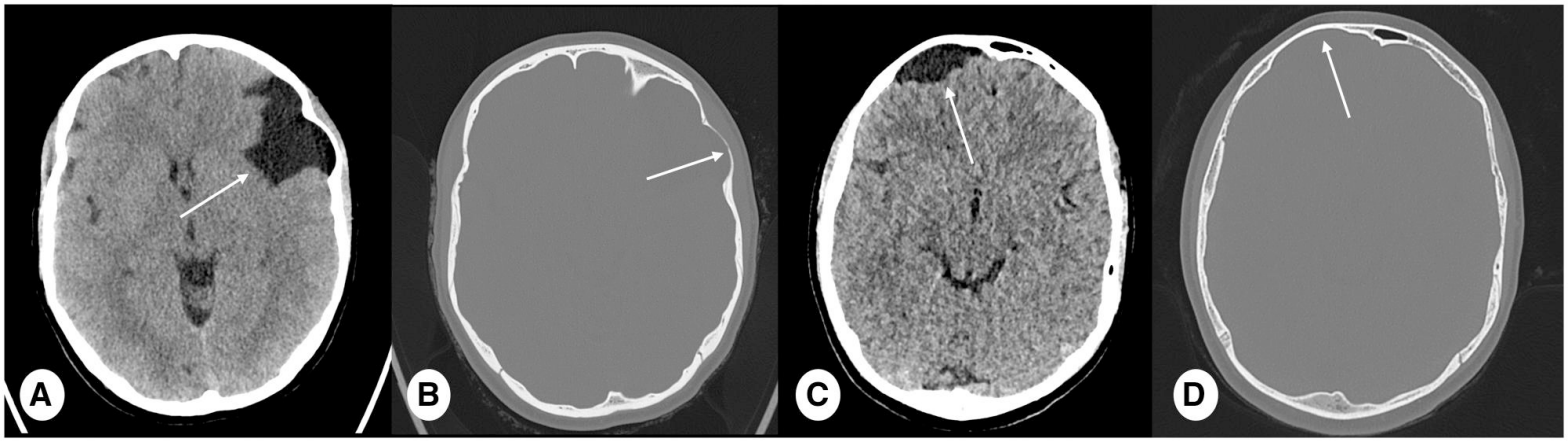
CT brain scan of a 17 year old female with arachnoid cyst (A, B). (A) PCD-CT ultra-high resolution CT (slice thickness 1 mm, kernel Hr40, and IR Q4) and (B) PCD-CT (slice thickness 1 mm, reconstruction kernel HR68, IR Q3). Corresponding EID-CT of a 12 year old male with arachnoid cyst in the brain (C, D). (C) Slice thickness 1 mm, kernel Hr40, IR level of 3, and (D) slice thickness 0.75 mm, kernel Hr60, and IR 3. (A) and (C) show an outline of arachnoid cysts in both patients, where the PCD-CT (A) image has a lower noise and sharper definition of the abnormality (arrows). Moreover, (B) and (D) (arrows) exhibit bone remodelling with thinning diploe bone wall. This comparison highlights the potential advantages of PCD-CT in achieving higher spatial resolution.
